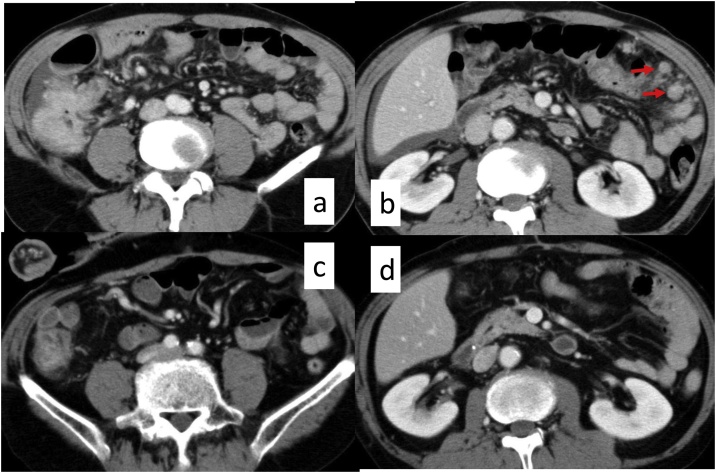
Thoracic–abdominal enhanced computed tomography (ECT). A, B. ECT showing the wall thickness of the ascending colon, ascites in the pelvic cavity and right upper abdomen, and suspected peritoneal disseminations (PDs) (arrow) in the left upper abdomen. C, D. After 18 courses of chemotherapy, the volume of the ascending colon cancer had shrunk to around 50% and peritoneal disseminations and ascites had disappeared.
